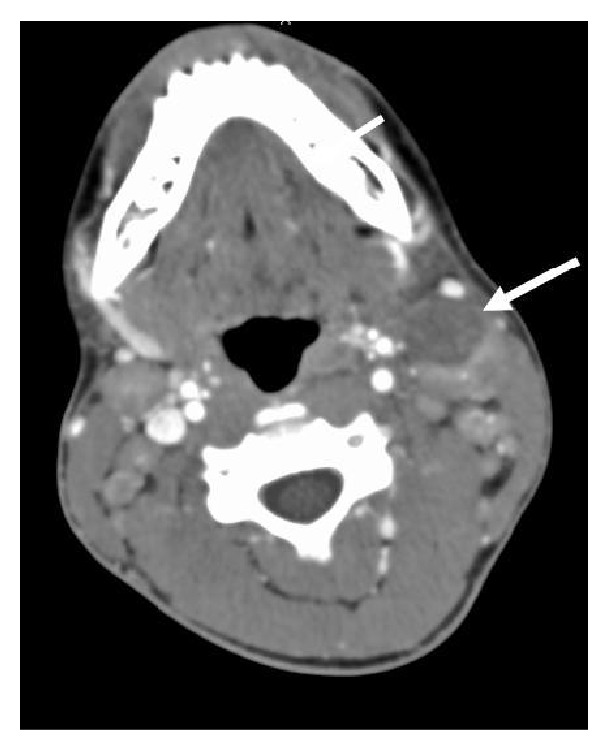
Axial CT angiogram showing bilateral cervical lymphadenopathy, the largest of which measures 4 × 3.4 × 2.5 cm (arrow). The posterior portion of the node demonstrates arterial phase enhancement while the anterior portion does not demonstrate it. This mixed density mass has the appearance of a necrotic or haemorrhagic lymph node.
